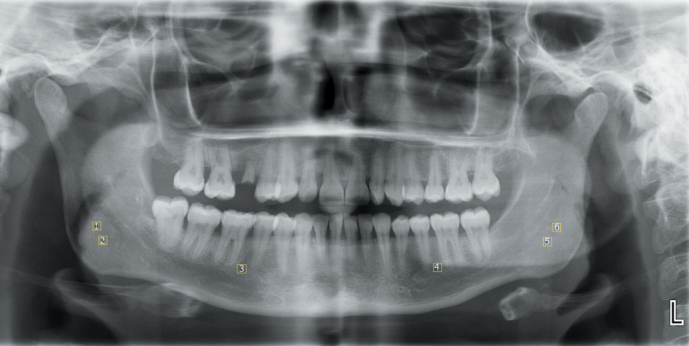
ROI selection on panoramic radiography to be used for FA. 385 × 194 mm (120 × 120 DPI)
